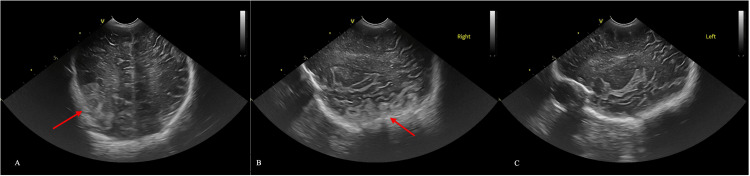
Cranial ultrasound images showing right parietotemporal hyperechogenicity in coronal view (A) and right parasagittal view (B); normal ultrasound images in left parasagittal view (C)
